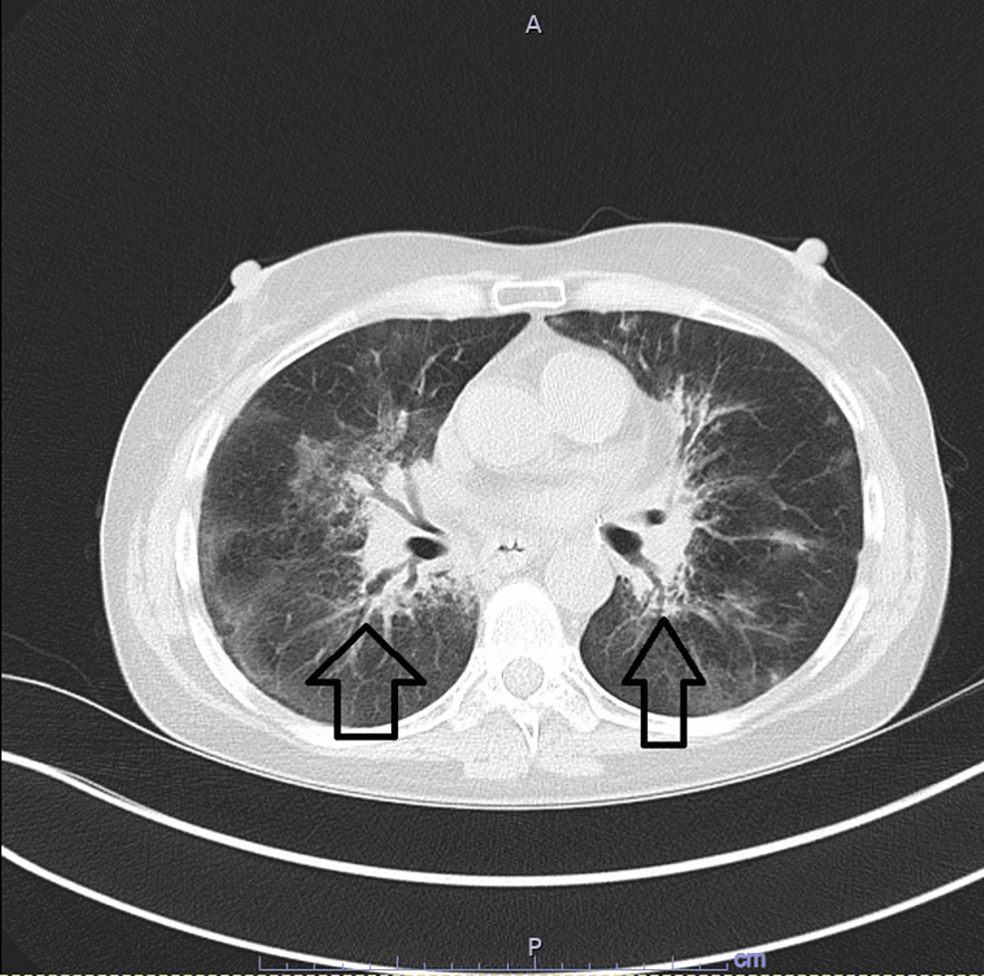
Improvement of Radiological CT Chest Findings A follow-up chest CT scan in January 2023 showed radiological improvement of infiltrative lung lesions (arrow).
